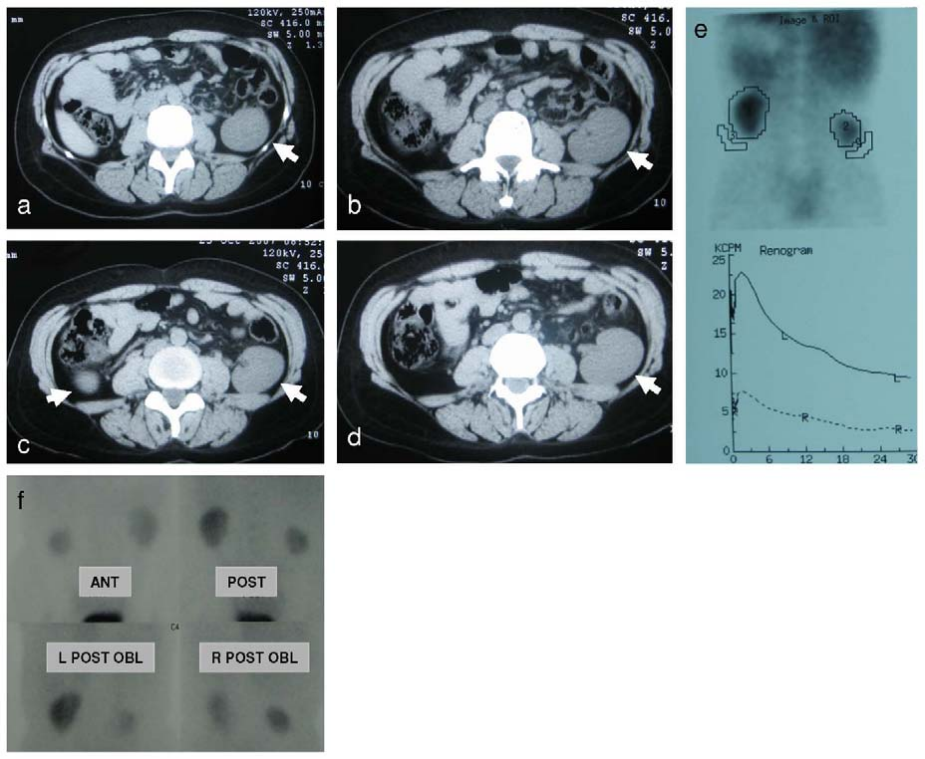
Hypoplastic and Hypofunctional Kidney in Human Lrp4 Mutations. CT scan reveals a severely hypoplastic kidney on the right and mild hypoplasia on the left side (a–d). Both kidneys are ectopic with caudal and lateral shifts (a–d). Dynamic-static renal scintigraphy with Tc-99m DTPA suggest right kidney dysfunction (e). Global renal functional participation; right kidney 26% and left kidney 74%. Static renal cortical scintigraphy with Tc-99m DMSA background activity of radiopharmaceutical is higher than expected (f).
